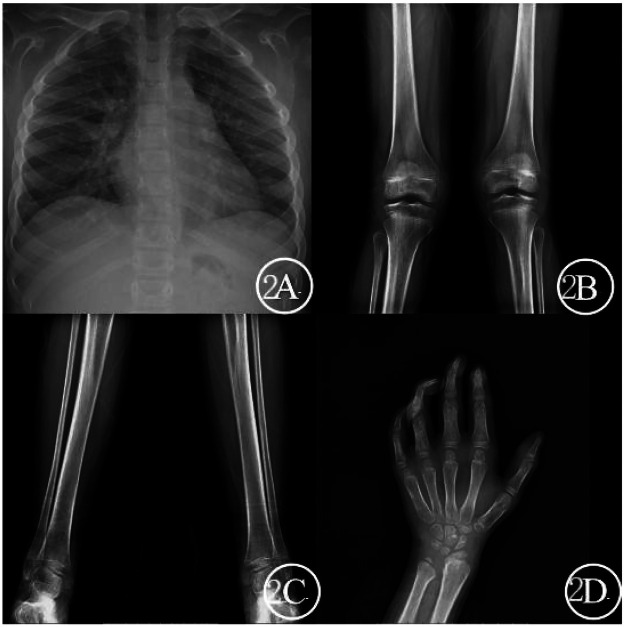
Pediatric patient radiographic examination (A) chest x-ray, anterior-posterior view; (B,C) full-length x-rays of both lower limbs, anterior-posterior view; (D) left hand x-ray, anterior-posterior view.
